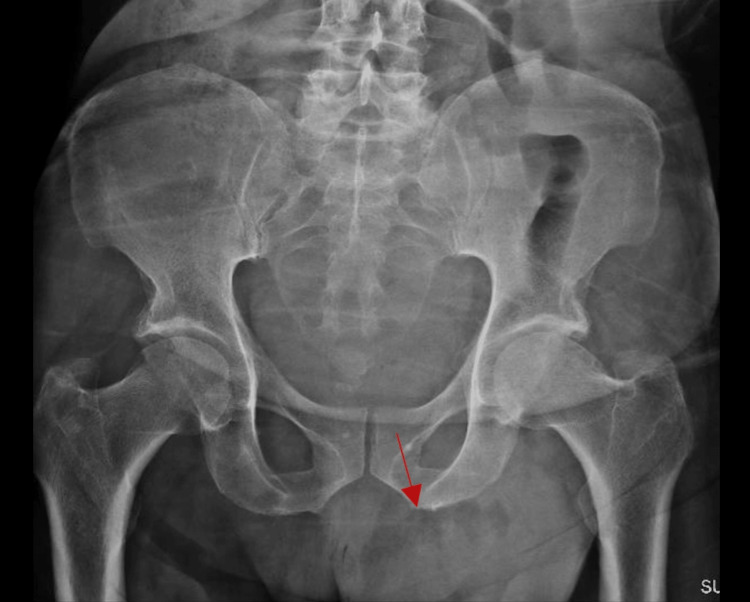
Pelvic X-ray showing small bowel in the left inguinal area (red arrow)
